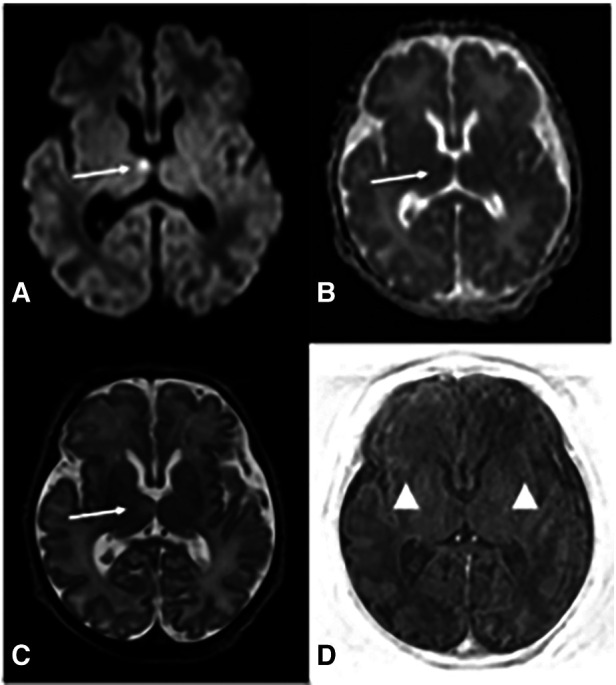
Brain MRI at d6 demonstrates deep gray and white matter hypoxia. Axial diffusion-weighted (DWI) image (A) reveals increased b1000 signal in the right ventro-medial thalamus and corresponding decreased apparent diffusion coefficient (ADC) (B) (*arrows in*
